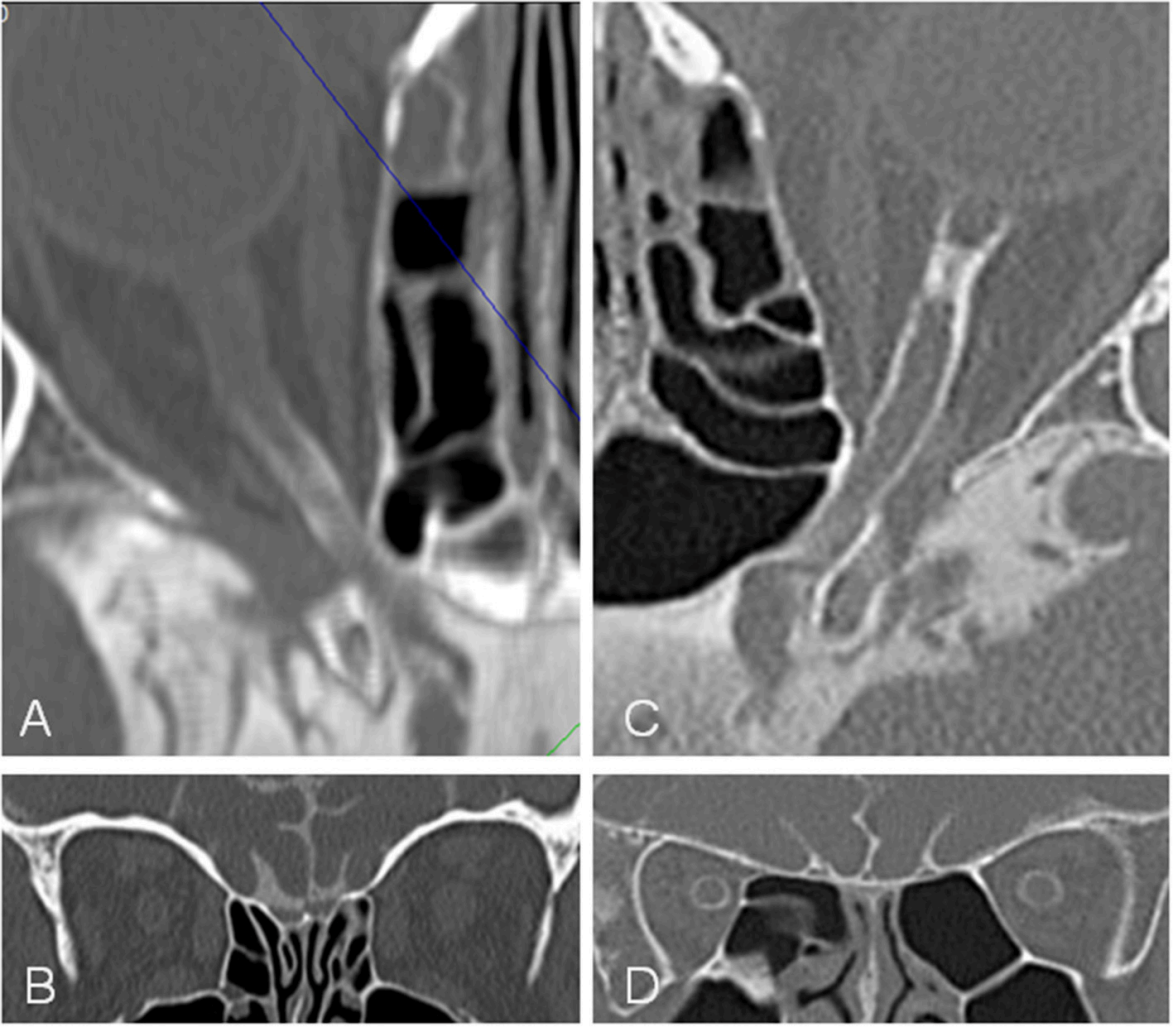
Computed tomographic (CT) cisternography in a patient with idiopathic intracranial hypertension (IIH) and papilledema and a control subject. In the patient with IIH (A,B) contrast loaded cerebrospinal fluid (CLCSF) inflow stops behind the optic canal while in the control subject (C,D) contrast loaded cerebrospinal fluid (CLCSF) flows into the orbital segment to the globe.
